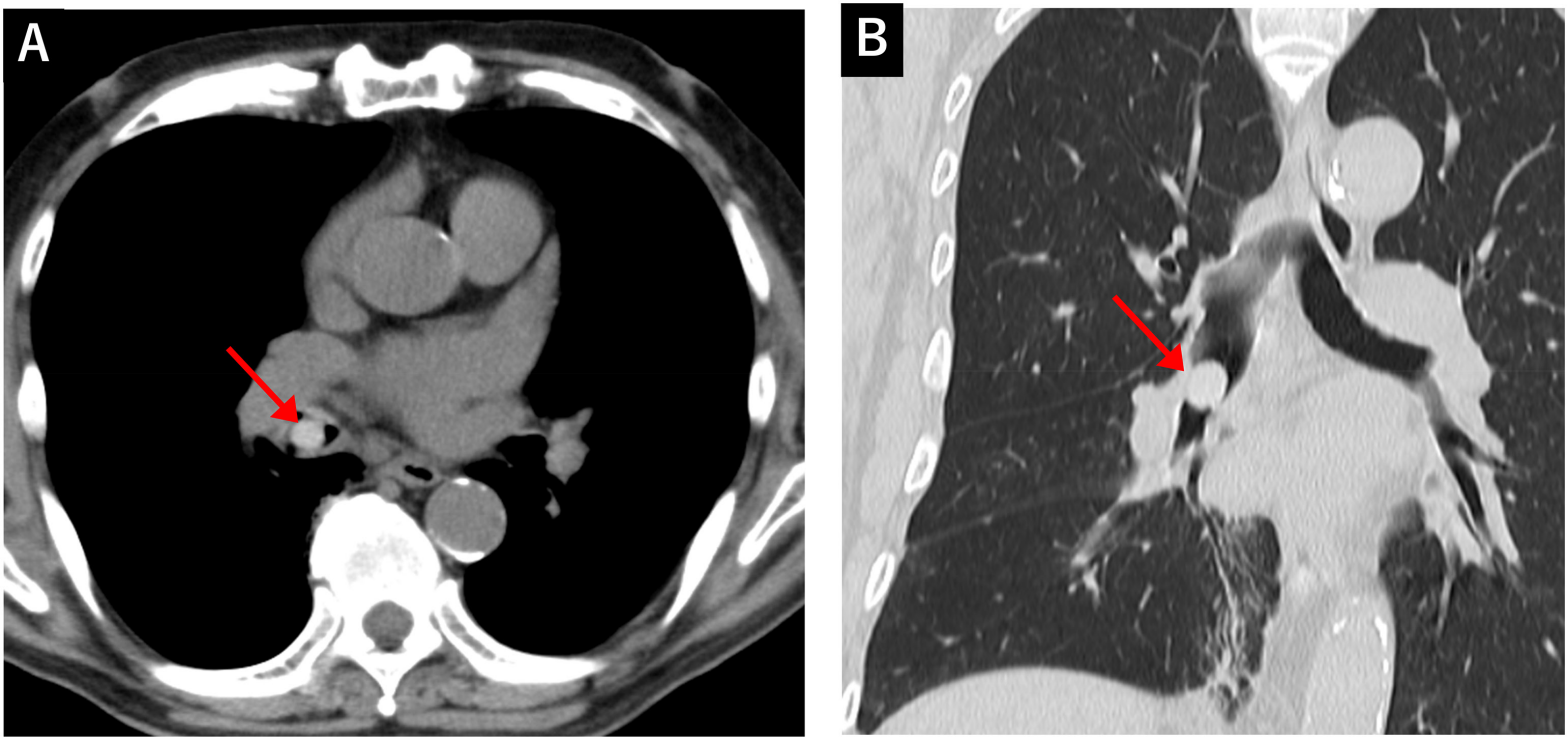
A non‐contrast‐enhanced chest computed tomography scan demonstrating a high‐density nodule in the right bronchus intermedius (indicated by red arrows). (A) Axial view with mediastinal window setting. (B) Coronal view with lung window setting.
